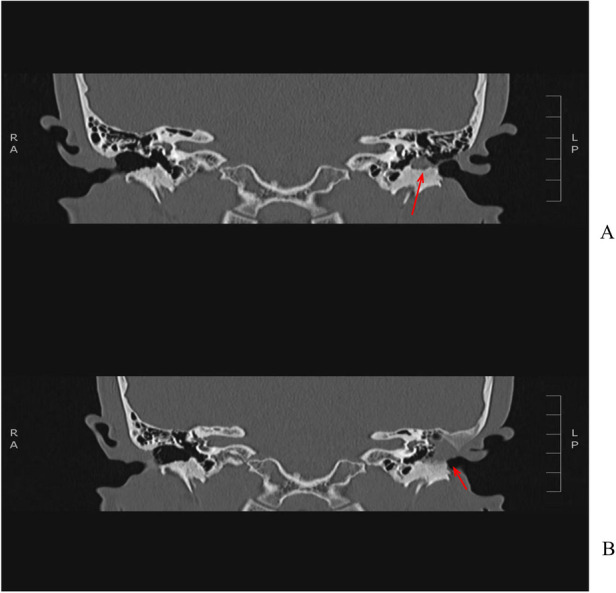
(A) (initial admission Ear CT findings) soft tissue density shadows with interspersed bony sclerosis are identified in the left external auditory canal, mastoid air cells, tympanic cavity, and mastoid antrum. The ossicles are embedded and show extensive bony destruction. (B) (Follow-up Non-contrast Ear CT Findings 8 Months After Left Mastoidectomy) Partial bony structures of the left mastoid and the entire left ossicular chain are absent. The residual mastoid bone exhibits sclerosis. Soft tissue density shadows are present in the tympanic cavity and mastoid antrum. The left external auditory canal is obliterated.
